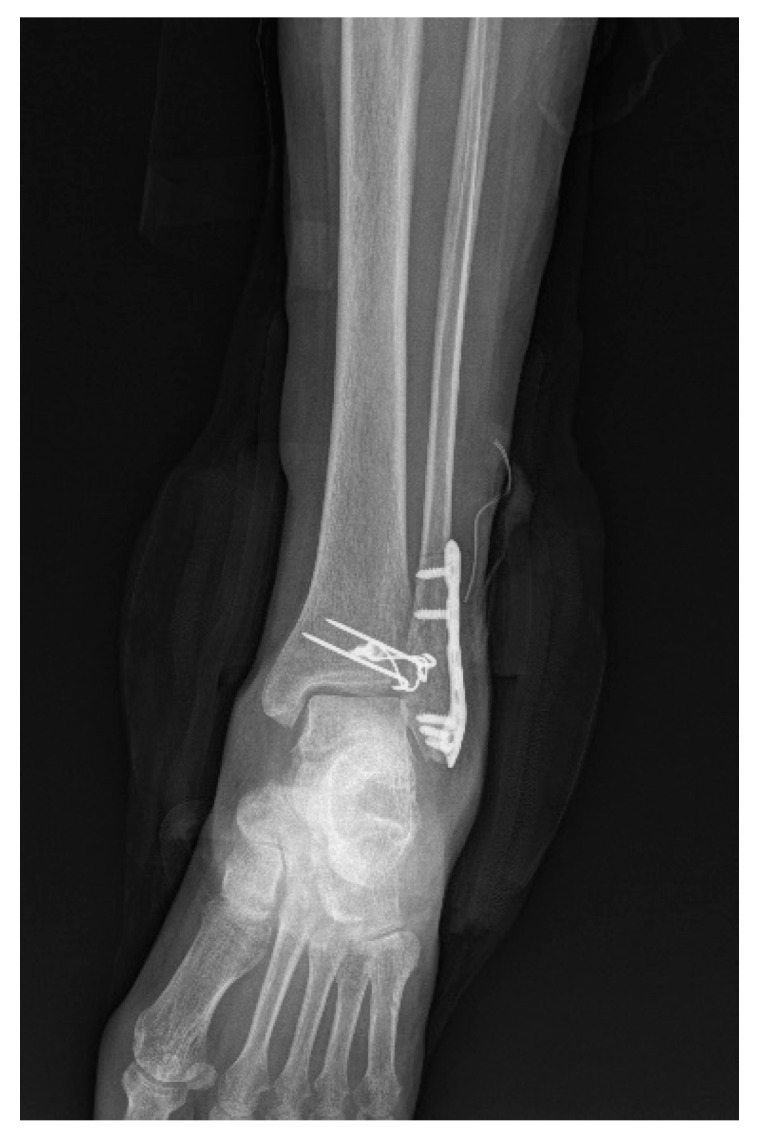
Postoperative X-ray of a Chaput fracture treated using the tension band wiring technique.
